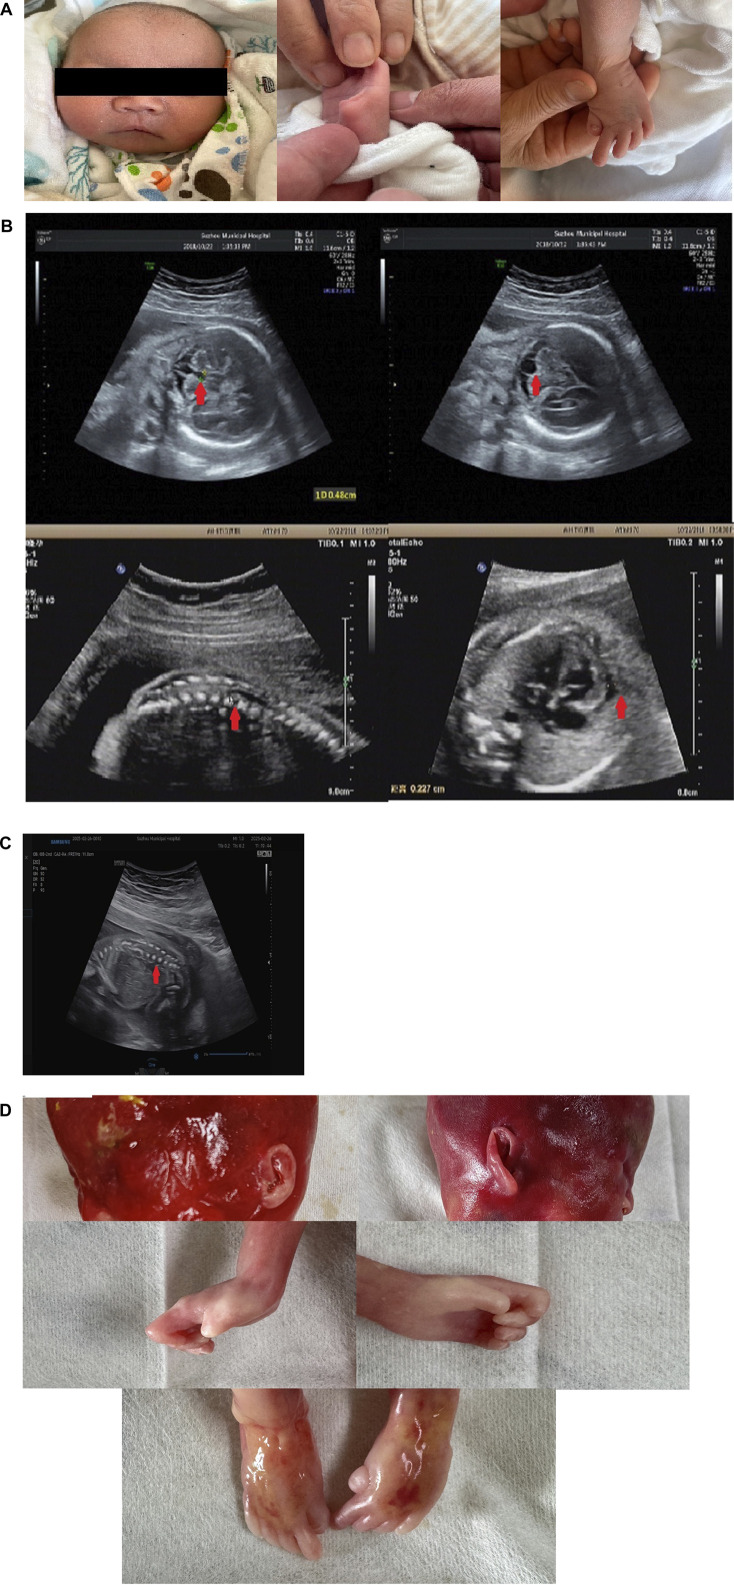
Phenotype of the patients: (A) facial malformation and thumb and toe anomalies of the proband. (B) Ultrasound scan of II-1 showed a dilated fourth ventricle, a posterior fossa cyst, a low conus medullaris position, and pericardial effusion. (C) Ultrasound scan of II-3 showed low conus medullaris position. (D) Low ear position and dysplasia of thumbs and halluces in II-3.
